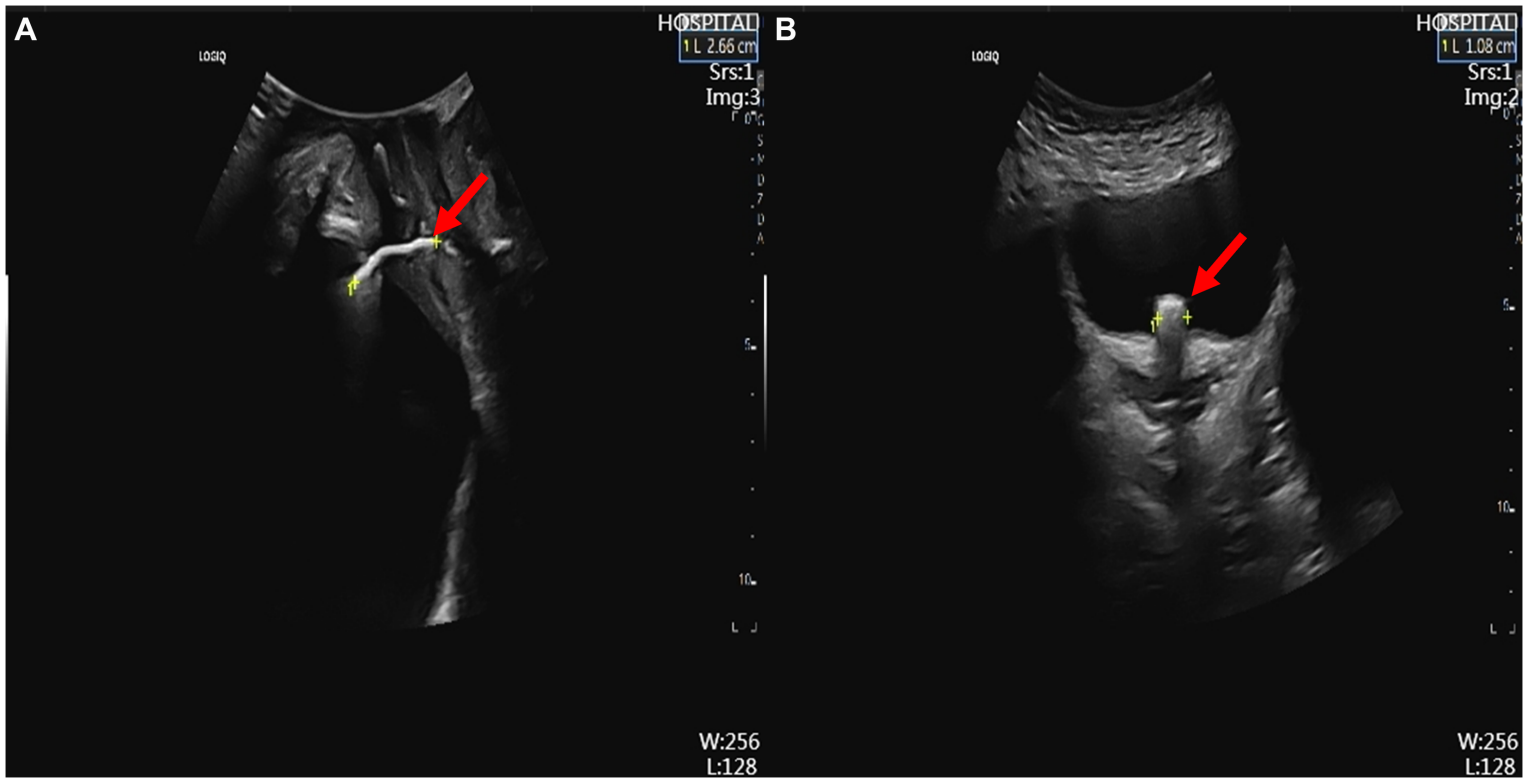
Ultrasound findings. (A) Transperineal ultrasound showing the IUD in the vesicovaginal space (red arrow). (B) Abdominal ultrasound showing one arm of the IUD penetrating the bladder wall, indicating bladder stone formation (red arrow). IUD, intrauterine device.
